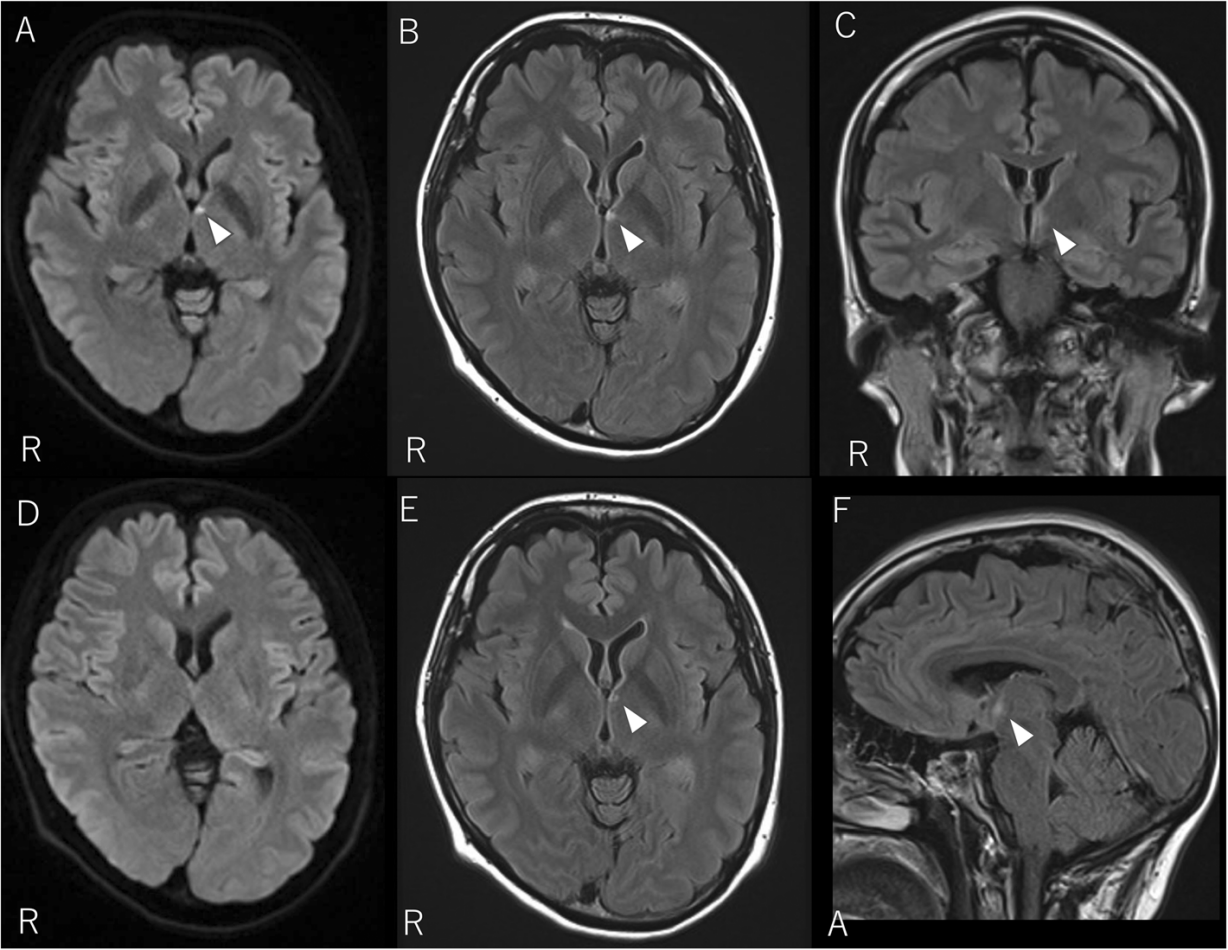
Brain MRI of the patient. Diffusion-weighted (a) and fluid-attenuated inversion recovery MRI images (b, c) obtained on admission reveal a small, high-signal intensity restricted to the left hypothalamus (arrowheads). Sagittal fluid-attenuated inversion recovery MRI shows involvement of the posterior part of the hypothalamus (f, arrowhead). Follow-up MRI after 3 months shows unremarkable findings on diffusion-weighted images (d) and reduced intensity of the hypothalamic lesion on fluid-attenuated inversion recovery MRI images (e) (arrowhead)
